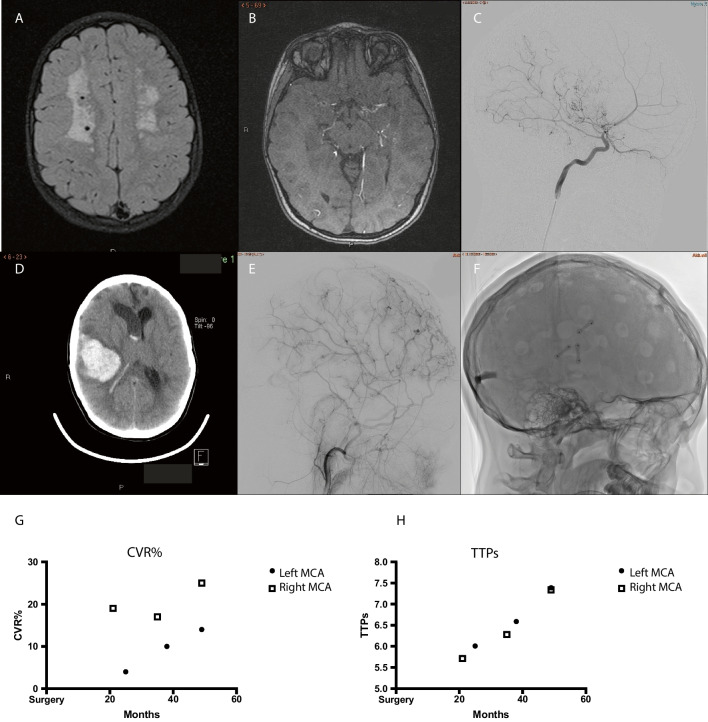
Patient 5 with bilateral MMD presenting at age 4. A Initial MRI showing chronic ischemia in white matter. B Follow-up MRI with time of flight sequence shows bilateral MMD, confirmed later with C DSA. At age 9, a revascularization procedure with multiple burr holes was performed. Three years after surgery, she developed an intracerebral hemorrhage on the right side D that was evacuated. A DSA was performed showing no aneurysms/AF fistulas. E Ingrowth of numerous blood vessels through the burr holes and improved perfusion were seen on DSA. F The location of the burr holes. G CVR was not established pre-op due to patient age. However, post-op we have been able to follow her CVR, which has generally been low, on the right side about 20% and on the left side between 4–14%. H TTPs has been low but has increased with time post-op
